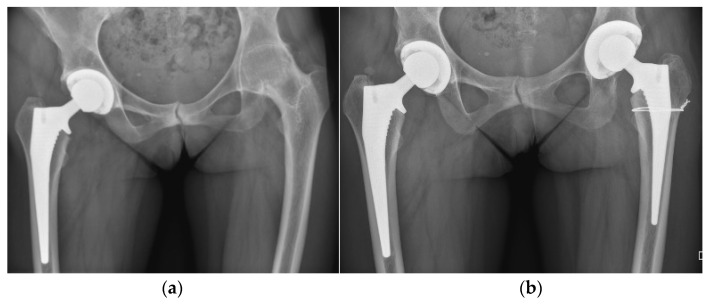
Secondary conversion of a hip arthrodesis to a total hip arthroplasty. (a) preoperative X-ray after left hip arthrodesis; (b) postoperative X-ray after secondary conversion to a total hip arthroplasty.
